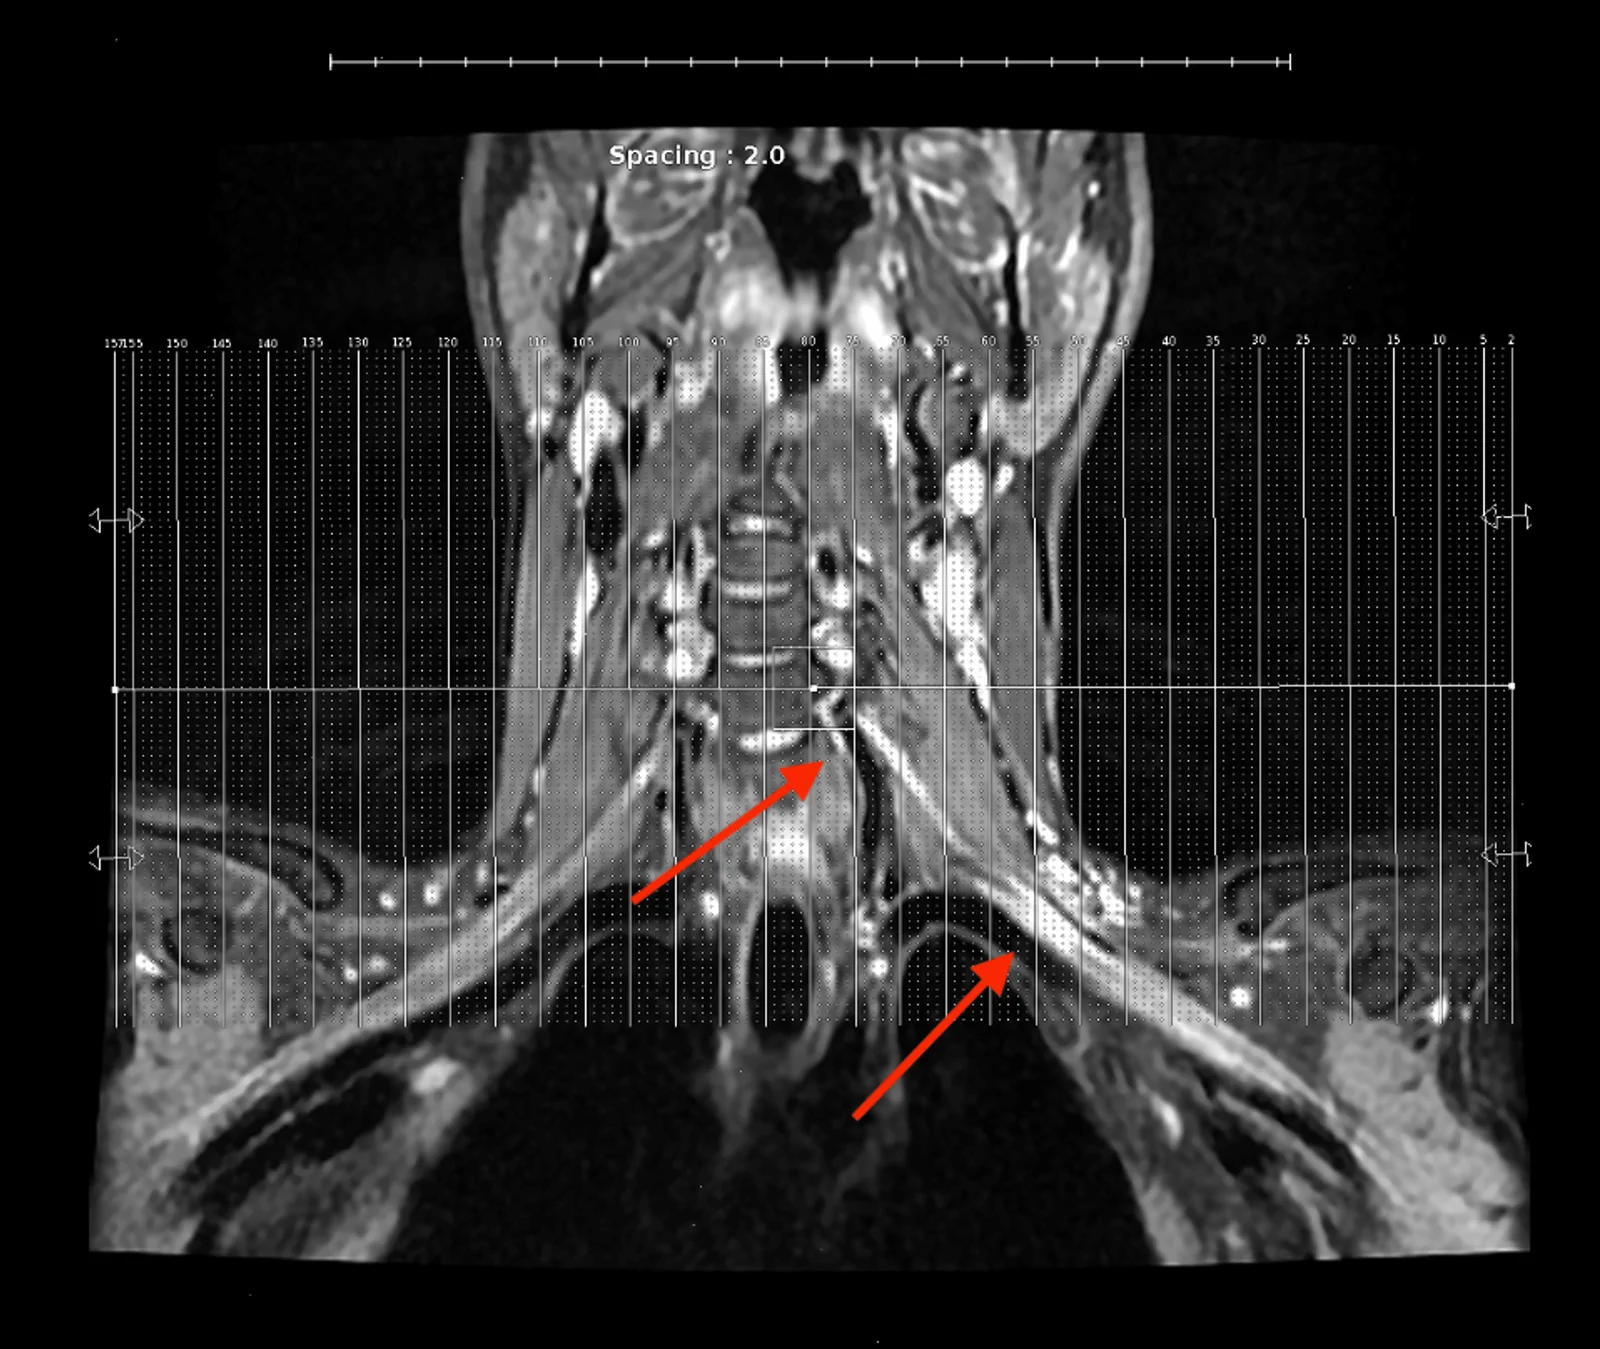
T2 cube coronal MRI L brachial plexus W/Wo contrast Enhancement, mild thickening, and nodularity of left brachial plexus, most prominently involving the left C5 and C6 nerve roots and left superior trunk.
